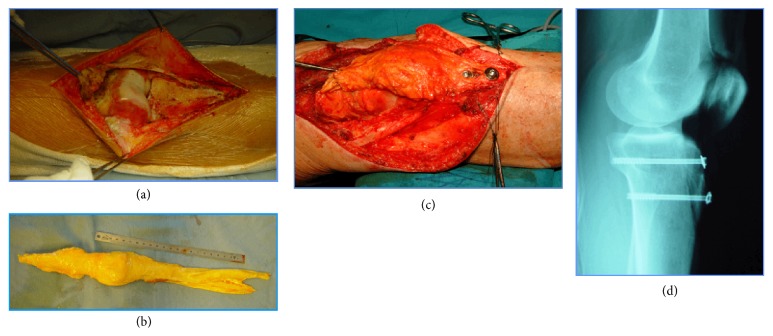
Surgical technique.* Clinical case (patient number 4) as example for the surgical technique used in the described study population. (a) Complete resection of lesion and extensor apparatus; (b) preparation of the osteotendinous allograft; (c) fixation of the allograft with screws distally and direct end-to-end suture proximally; (d) postoperative radiography.
